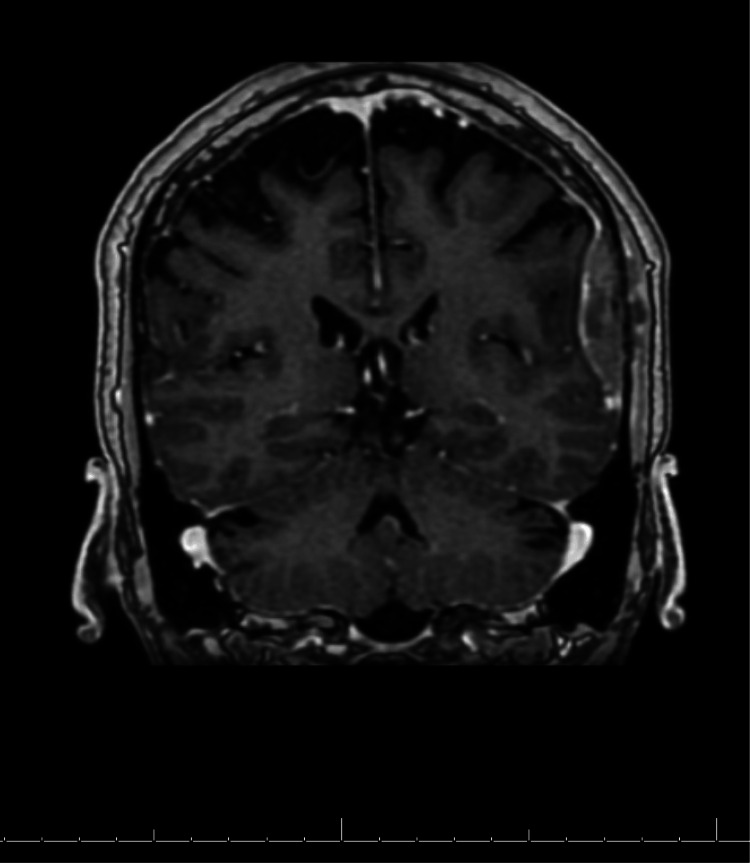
MRI brain, coronal view, showing dural-based lesions in the mid posterior left temporal lobe measuring 1.2 x 4.5 cm
